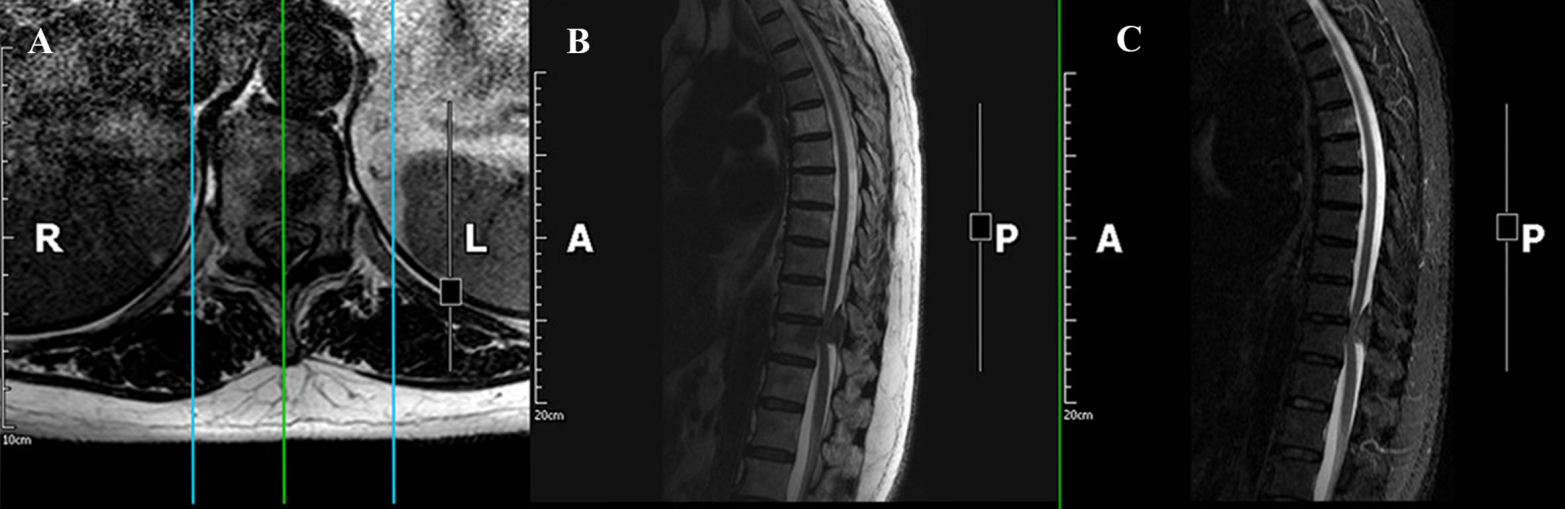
MRI demonstrated a mass between the T10 and T11 levels. (A) Axial T2-weighted image; (B) Sagittal T2-weighted image; (C) Sagittal T2-weighted image with fat-suppression.
